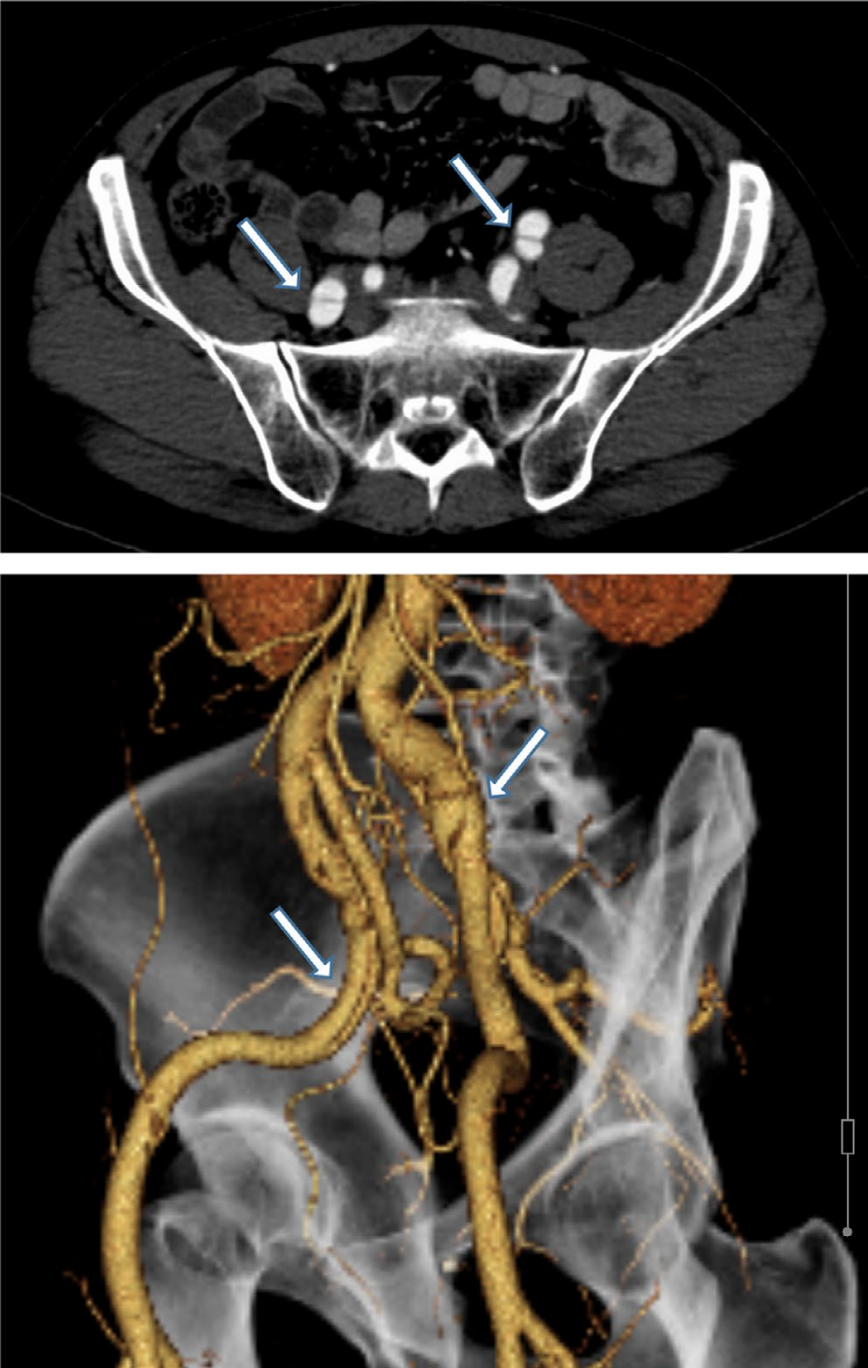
Computed tomography demonstrating bilateral external iliac artery dissection (arrows)
